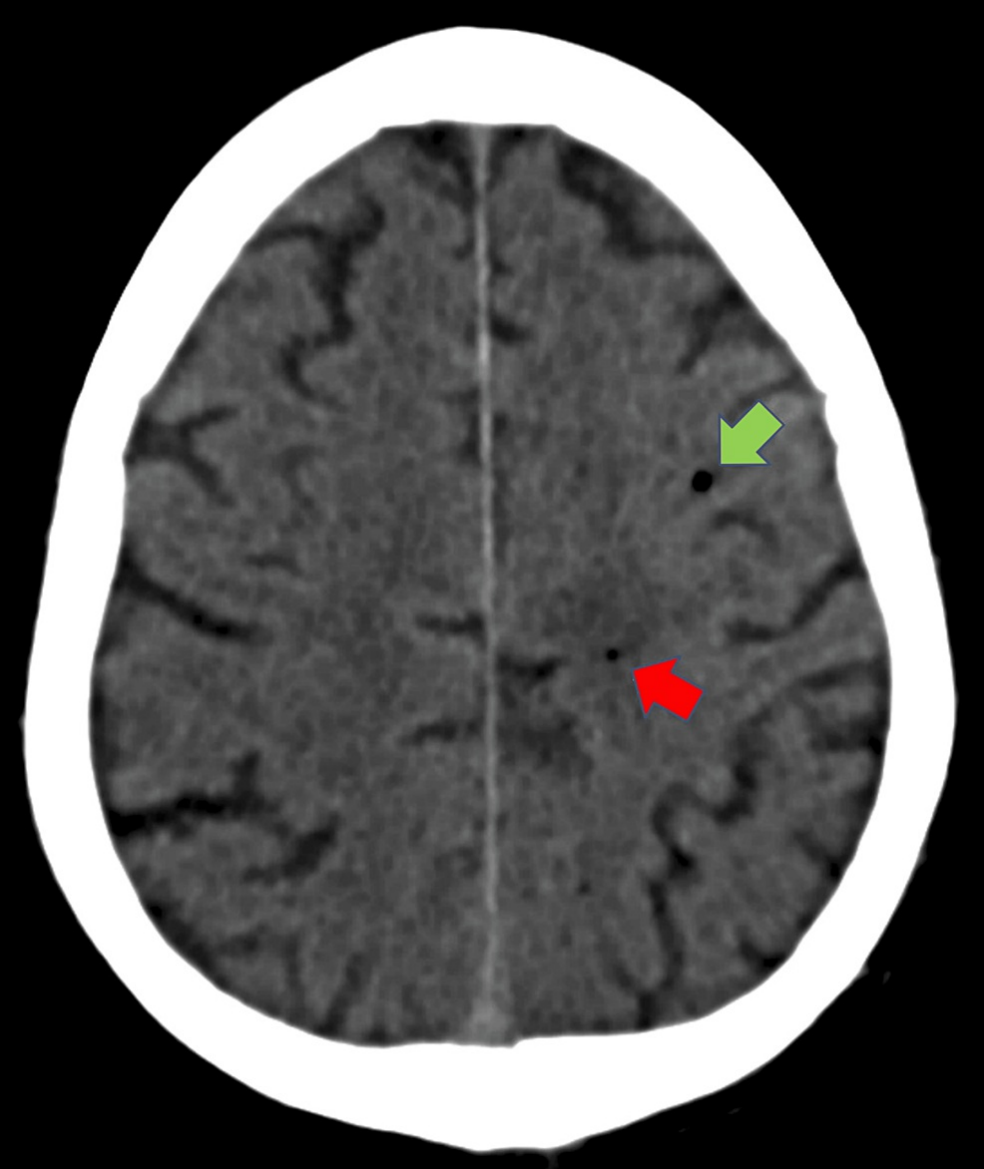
Unenhanced axial computed tomography (CT) brain demonstrating a few isolated air locules (green and red arrows) predominantly around the left anterior cerebral artery (green) – middle cerebral artery (red) watershed region.
